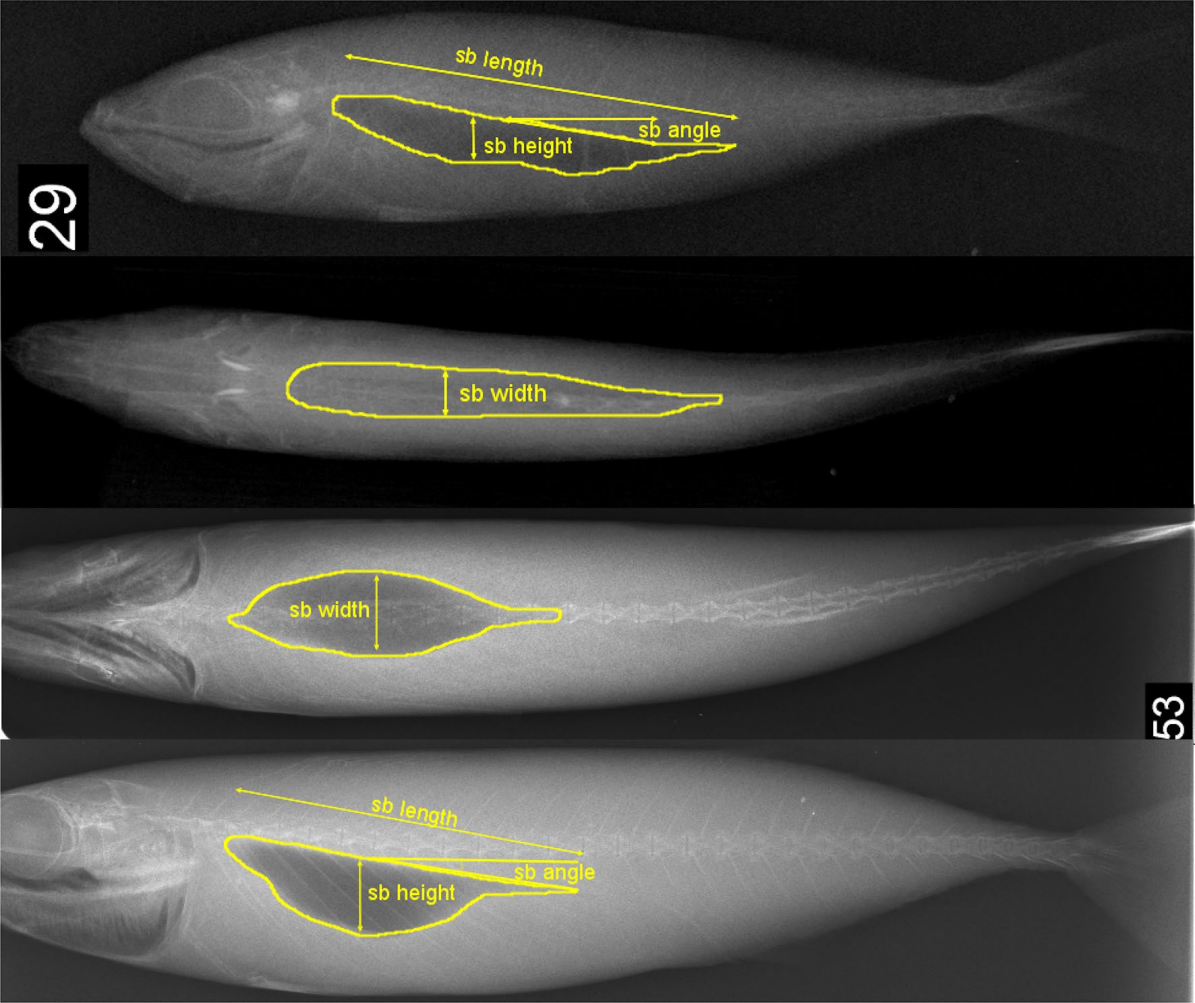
Soft X-ray lateral and dorsal radiographs of *T. mediterraneus* (a) and *S. colias* (b). Swimbladder borders are shown with yellow lines, while the arrows depict the swimbladder measurements.
